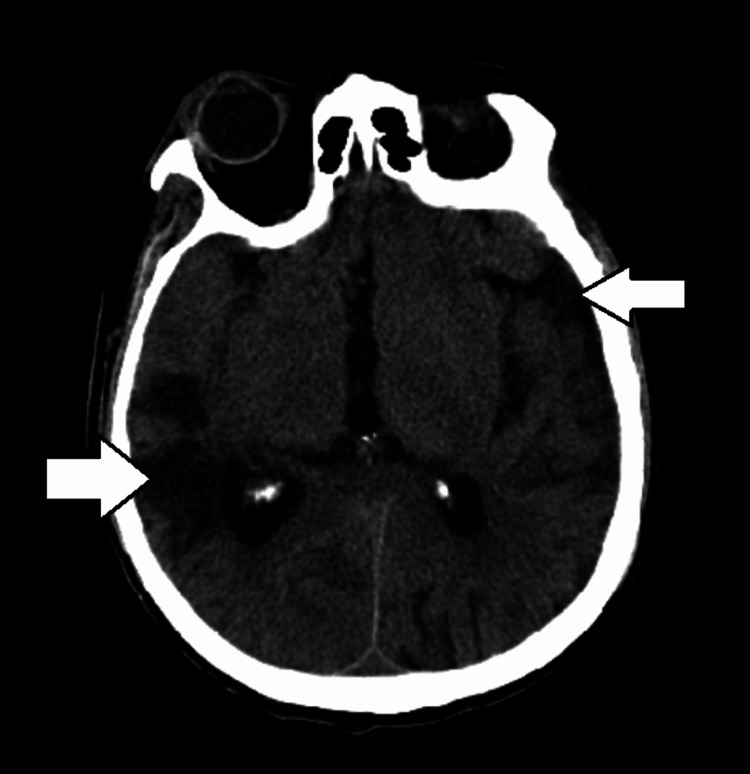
CT scan of the head without contrast showing diffuse encephalomalacia (white arrows).
